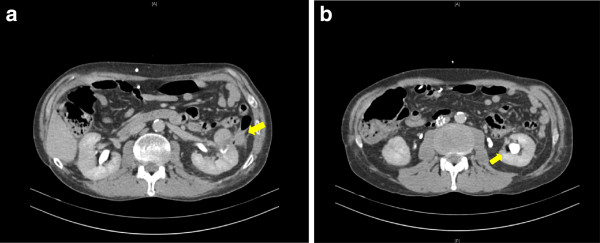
(A, B) Computed tomography scan of the colorenal fistula at presentation shows bubbles of gas in the area of stranding and the left collecting system
